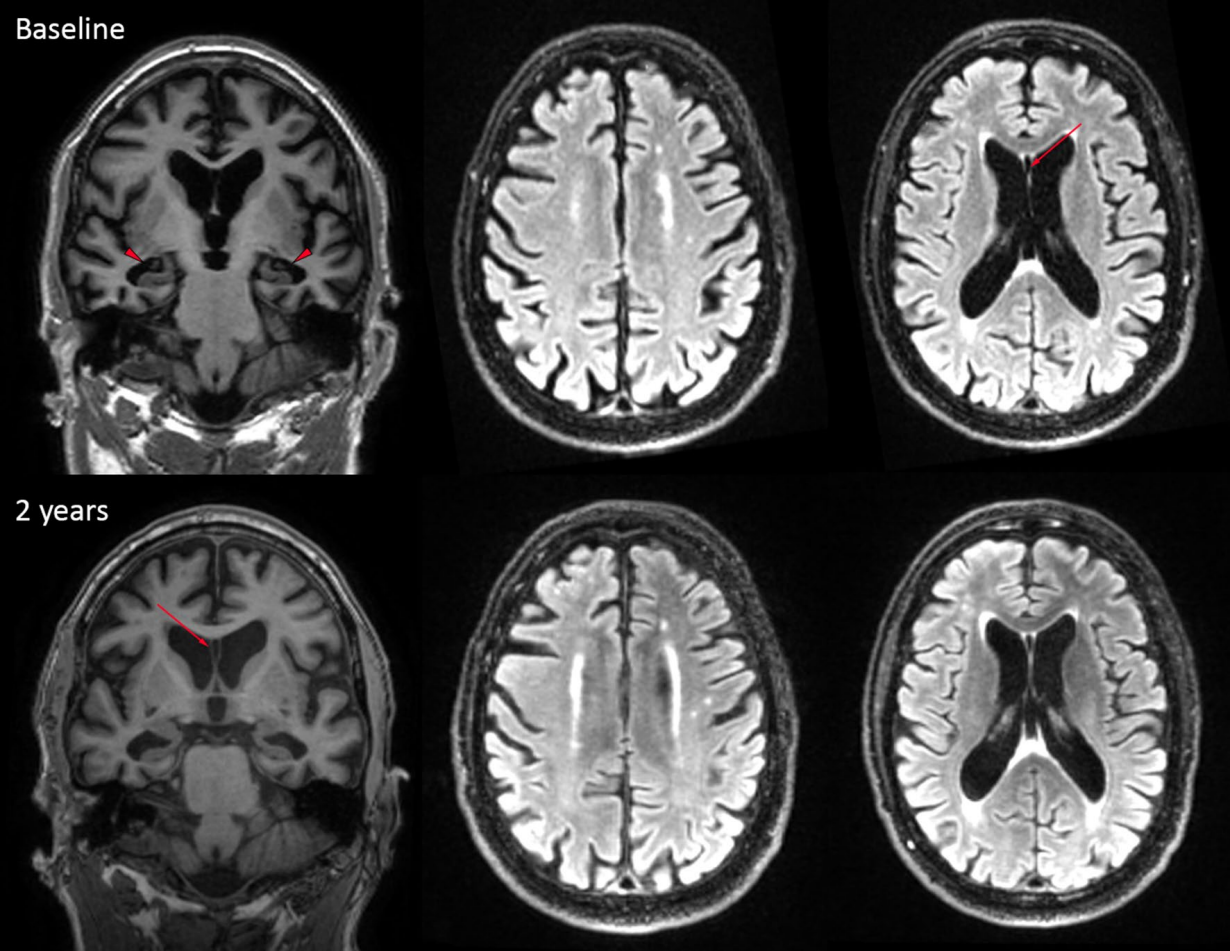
Brain MRI. Images were acquired at baseline and after two years. The coronal T1 images on the left show global atrophy (global cortical atrophy score 1–2), and severe bilateral hippocampal atrophy (medial temporal atrophy score 3, red triangle). The axial T2 FLAIR images in the middle and on the right display the white matter hyperintensities. The red arrows indicate the cavum septum pellucidum (CSP)
